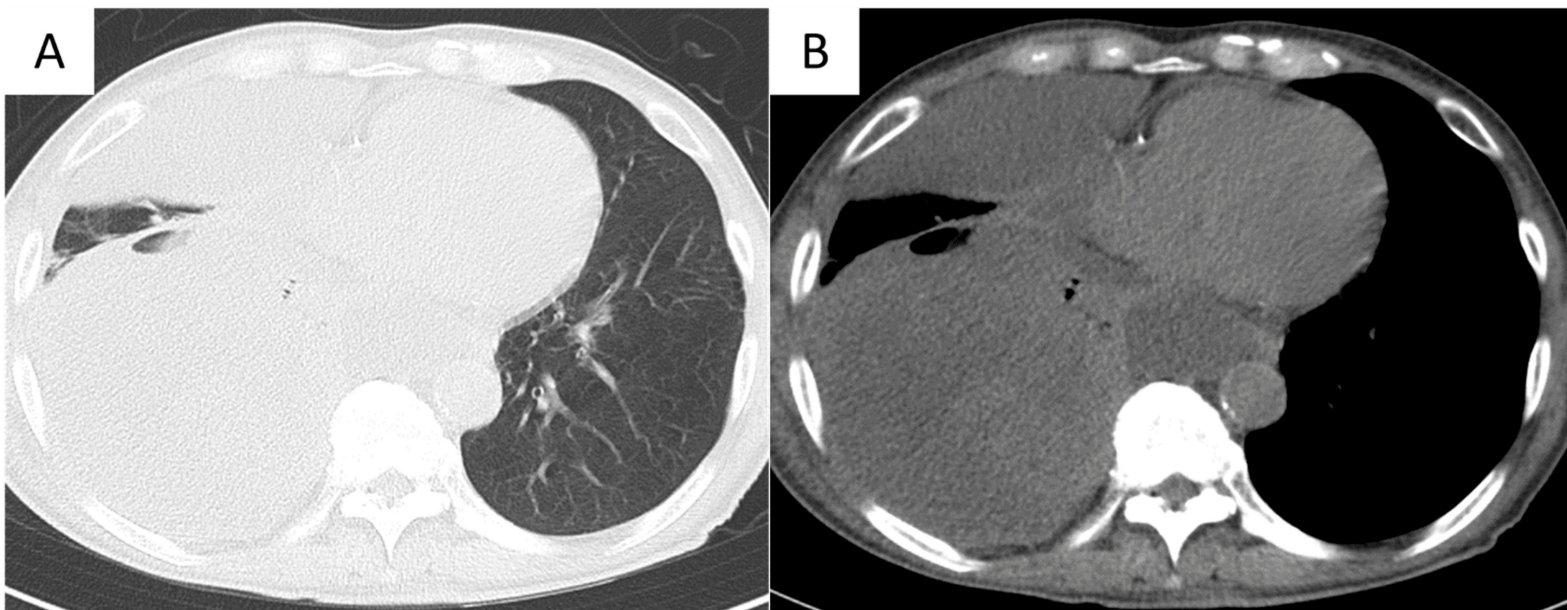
Chest computed tomography findings of chest at admission to our hospital Non-contrast chest computed tomography scan at admission to our hospital showed the right lower lobe lesion had increased in size, up to 110x110x150 mm, with fluids within the mass. A: pulmonary window, B: mediastinal window
